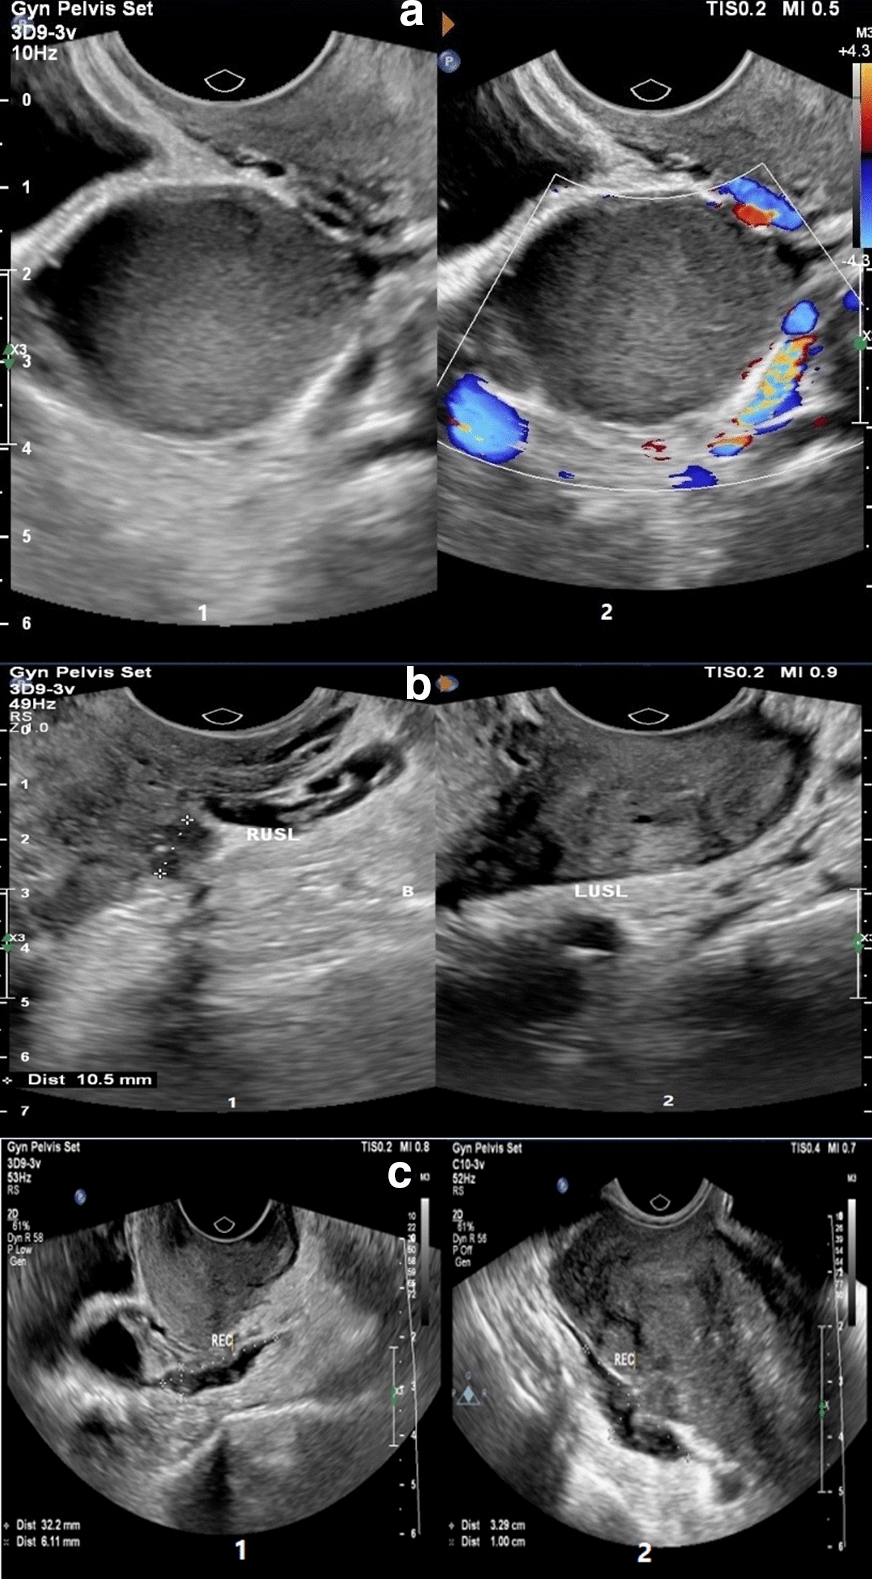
a Typical ovarian endometrioma in a woman with long-standing chronic pelvic pain and dysmenorrhea (1, 2) gray-scale (1) and color Doppler (1) TVS images of right ovary demonstrate a unilocular cyst containing homogeneous low-level echoes and no internal vascularity at color Doppler US (classic appearance of an ovarian endometrioma). b USL DIE in a woman with severe pelvic pain and dyspareunia for 10 years with a history of stage IV endometriosis who was confirmed to have extensive endometriosis at laparoscopy. (1) sagittal gray-scale TVS image shows irregular thickening of the right USL associated 10 mm endometriosis nodule in proximal. (2) Also, a moderate thickening of left USL has been shown. c Bowel DIE in two women. (1) Sagittal gray-scale TVS image in a woman with severe dysmenorrhea shows a hypoechoic nodule involving the serosal layer in the lower rectum. (2) Transverse gray-scale TVS images in a woman with chronic pelvic pain and cramping, show a hypoechoic nodule in the rectosigmoid junction with severe adhesion to the posterior of the uterus fundus
